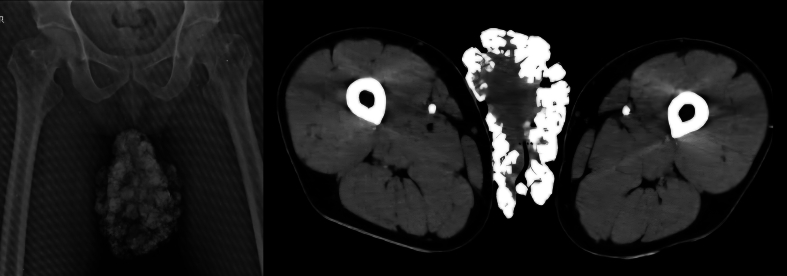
Radiological findings. 2a Xray, 2b CT scan (Multiple calcified lesions on scrotum).
